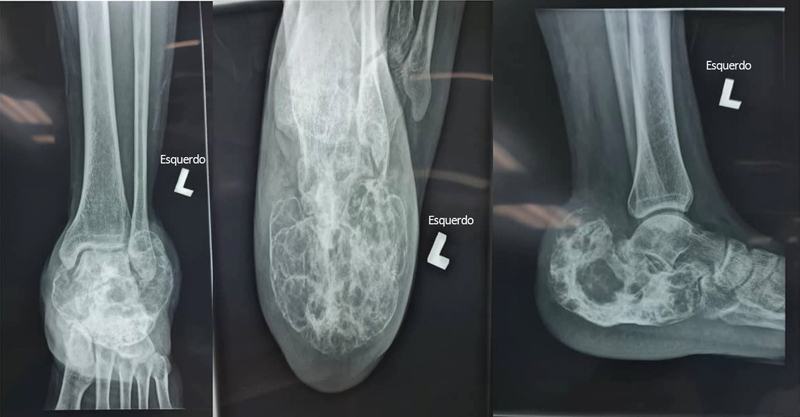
Radiograph imaging of the L pathological calcaneum in (
